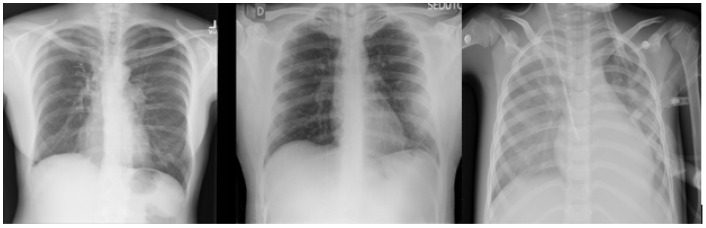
X-ray images of Normal, COVID-19, and Pneumonia used in binary classification task.
